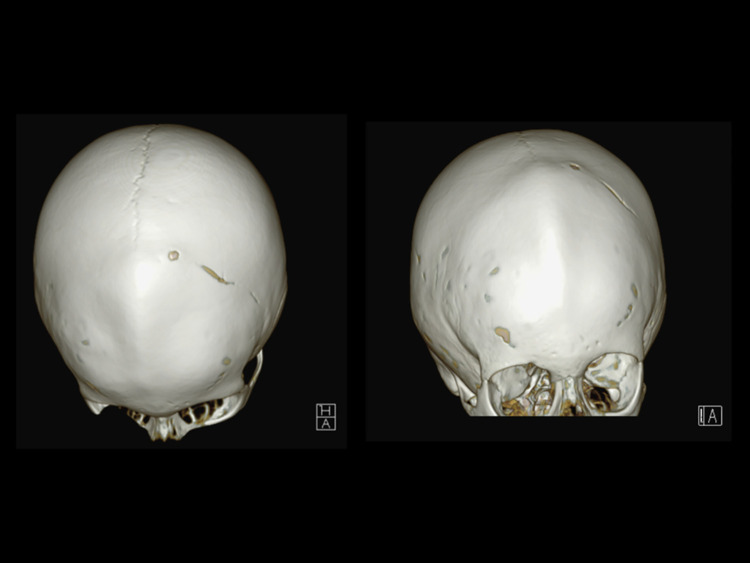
Computed tomography 3D images of the skull of the Family 1 proband, showing the craniosynostosis due to the precocious closure of the metopic and coronal sutures.
